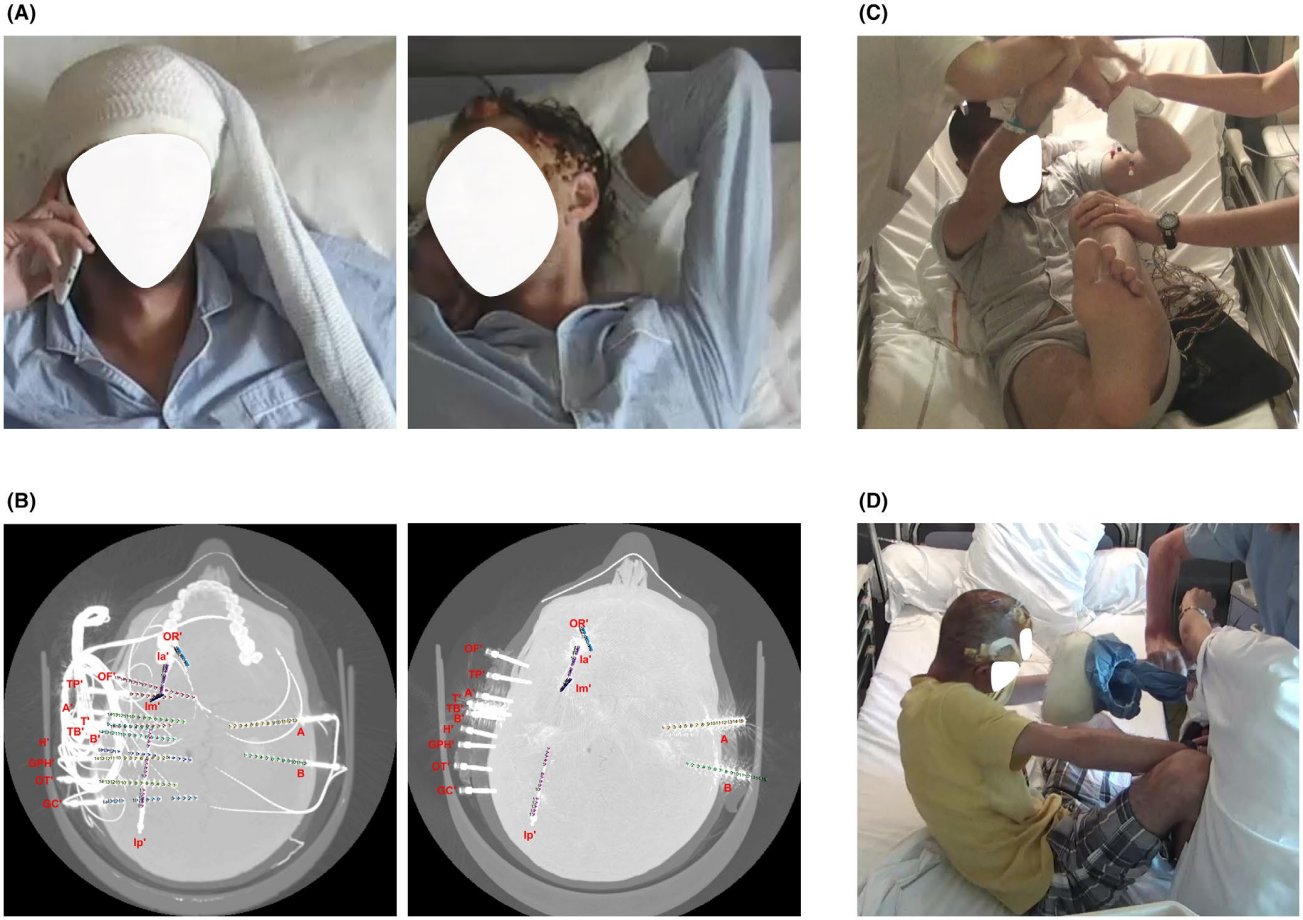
Behavioral and imaging aspects of postictal self‐electrode removal during SEEG monitoring. (A) Patient 1 before (left) and after (right) self‐removal of intracerebral electrodes. (B) Post‐implantation (left) and post self‐removal (right) head computed tomography scans of Patient 1. After self‐extraction, only the anchors of the left electrodes with orthogonal trajectories are visible, while the oblique and right‐sided electrodes appear displaced. (C) Patients 2 and (D) Patient 3 during the postictal period exhibiting agitation and resistive behavior while pulling their electrodes, as nursing staff intervene.
